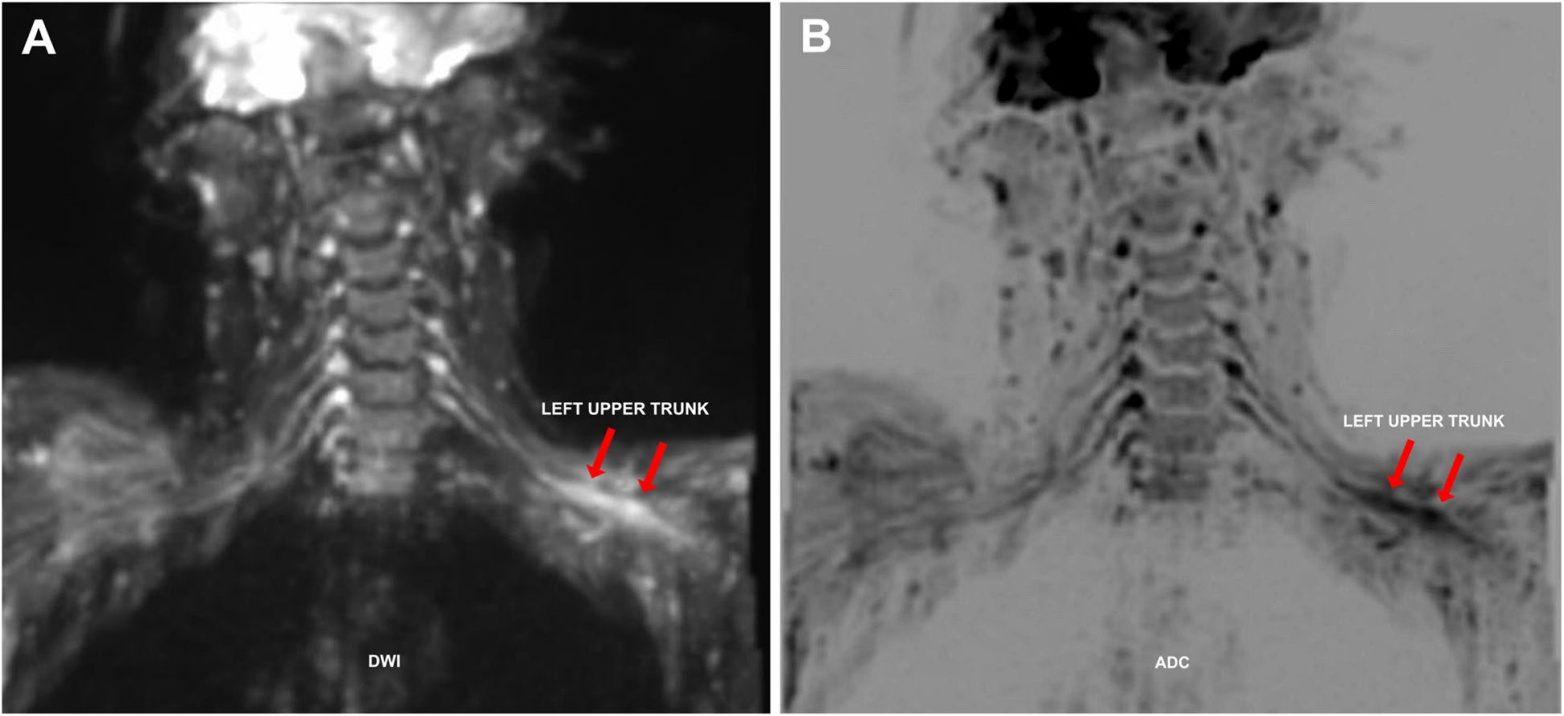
(A-B): DWI coronal scan showing segmental DWI-restriction of the left upper trunk (proximal–distal extension:6 cm) (A), confirmed at ADC-map (B)
